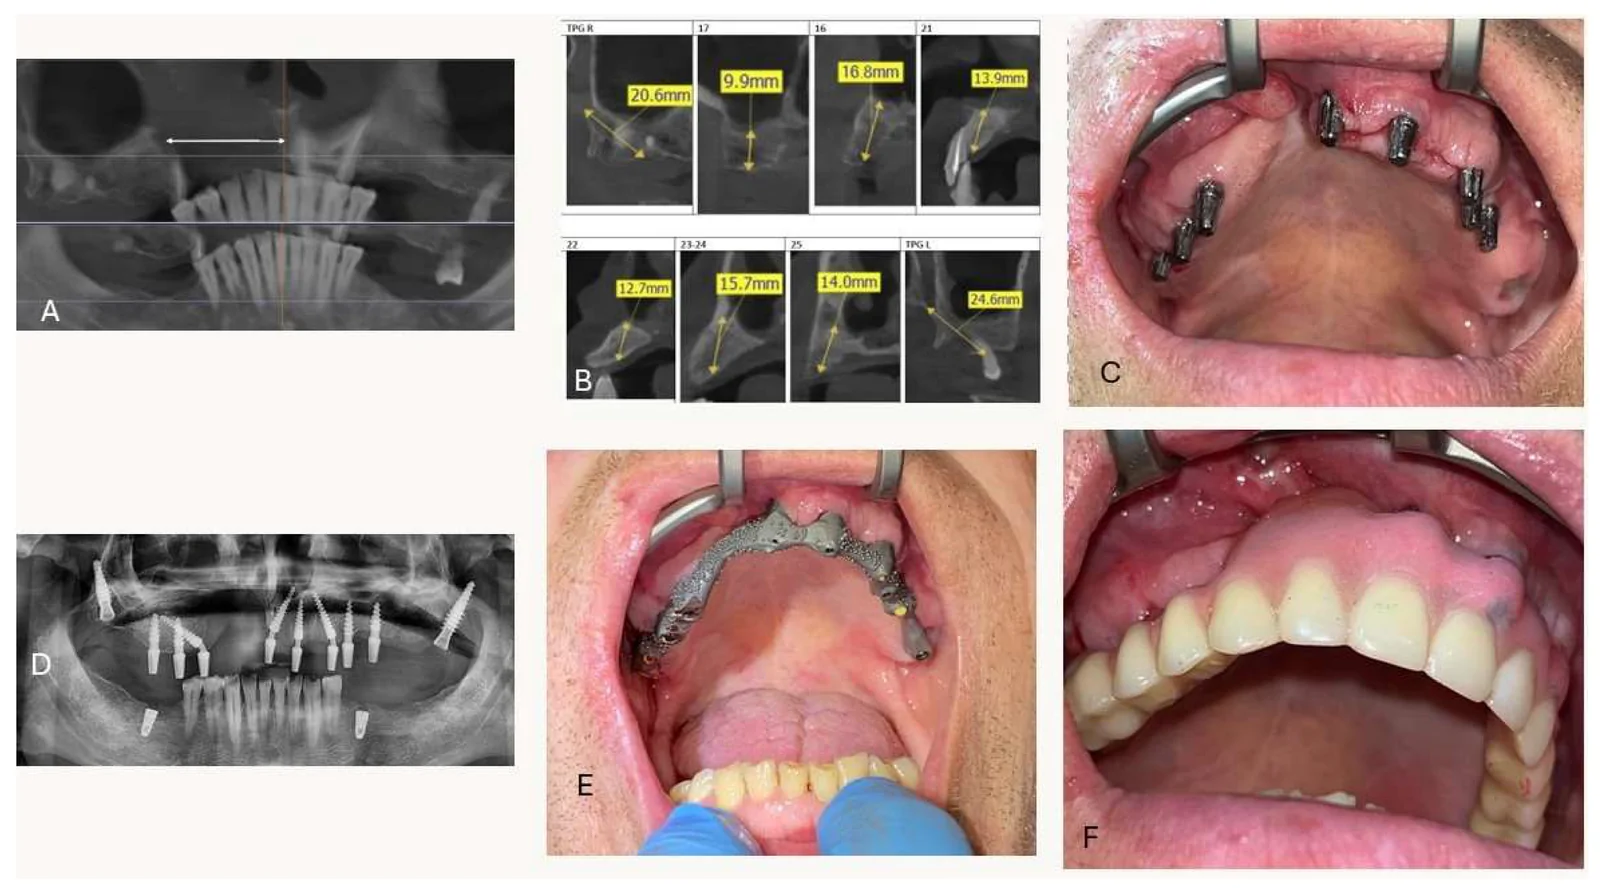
Clinical and radiographic management of Case 1. (A) Pre-operative CBCT screen with visualization of the maxillary defect. (B) Measurements of the height of the alveolar process in potential implant sites. (C) Intraoral view after removal of 18, 14, 21, 23, and 28 teeth, bone reduction in maxillary frontal area and placement of 10 implants. (D) Post-operative panoramic X-ray with visualization of implant location. (E) Intraoral view. Framework try-in. (F) View of the hybrid prosthesis in the mouth.
